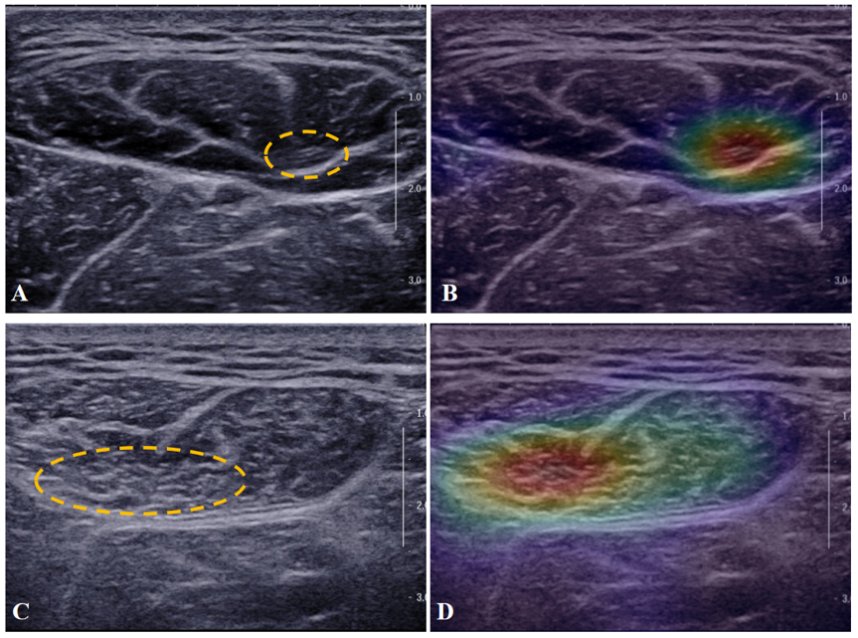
The corresponding heat maps of ultrasound images of the SARCO model. (A and B) The corresponding heat maps of ultrasound images of a participant without sarcopenia. (C and D) The corresponding heat maps of ultrasound images of a participant with sarcopenia. High activation regions are marked with dotted lines.
